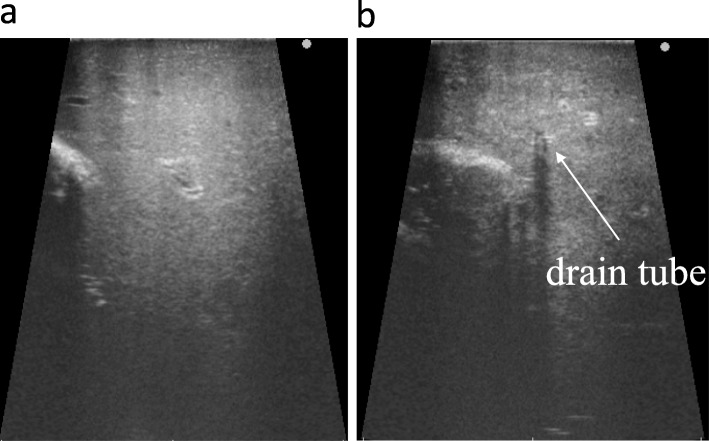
Photographs of intraoperative ultrasonography. a The normal liver, and b the necrotic liver and drain tube
